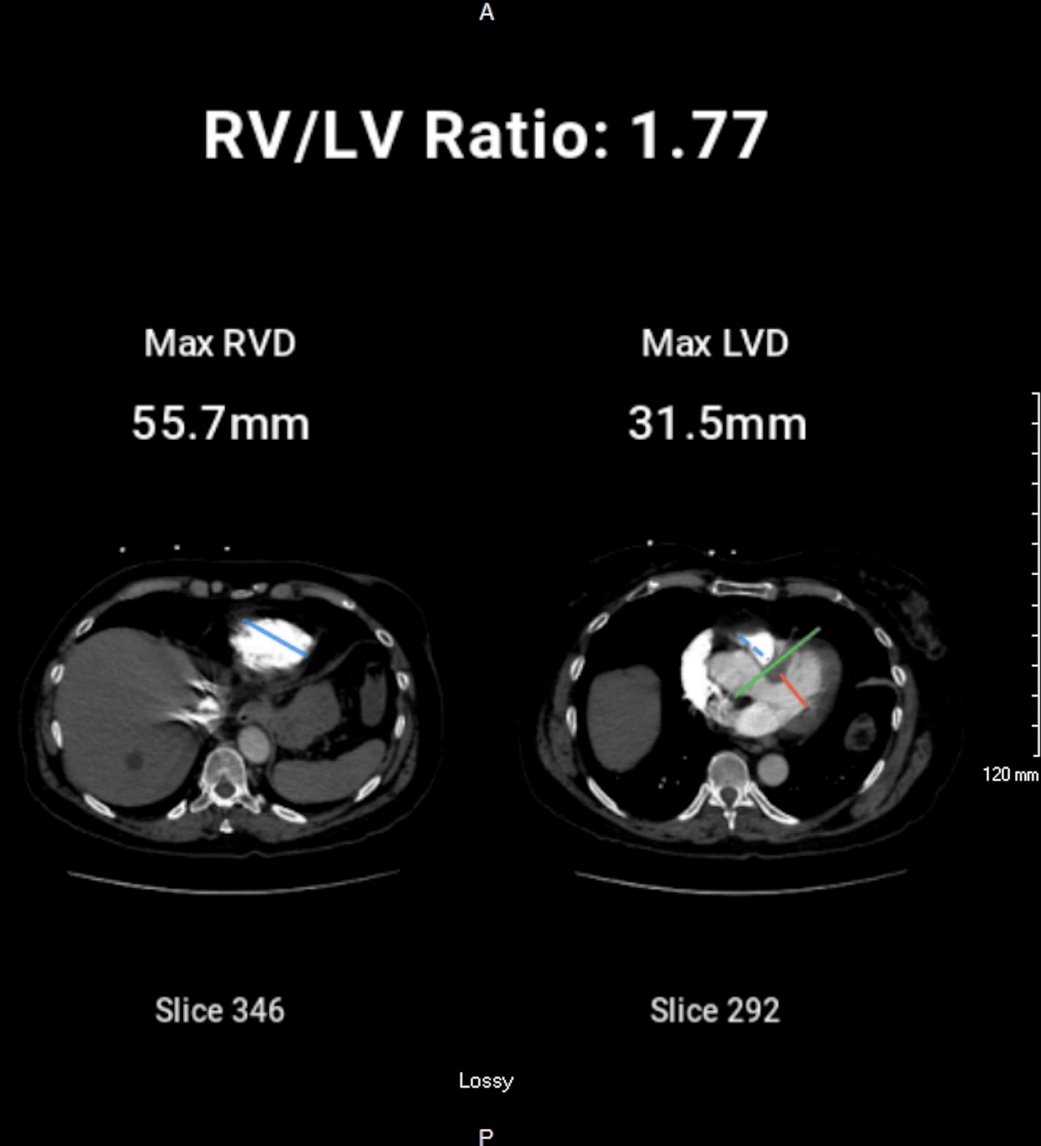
Maximal ventricular diameters on CTA. Left panel (Slice 346): Blue line indicates the maximal transverse diameter of the right ventricle (55.7 mm). Right panel (Slice 292): Red line shows the maximal transverse diameter of the left ventricle (31.5 mm), blue line marks the corresponding right ventricular diameter in the same slice, and the green line denotes the interventricular septum. The resulting RV/LV ratio of 1.77 reflects significant right ventricular enlargement. CTA: computed tomography angiography.
